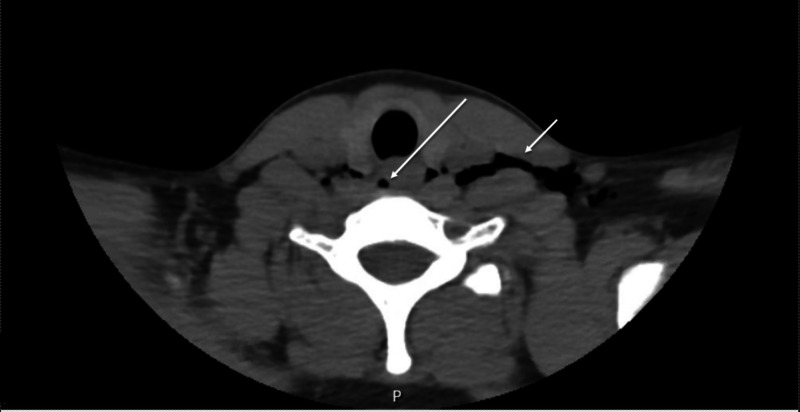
CT of the neck demonstrating subcutaneous emphysema (hypodense gas denoted by the right white arrow) and retropharyngeal emphysema (hypodense gas denoted by the left white arrow)
